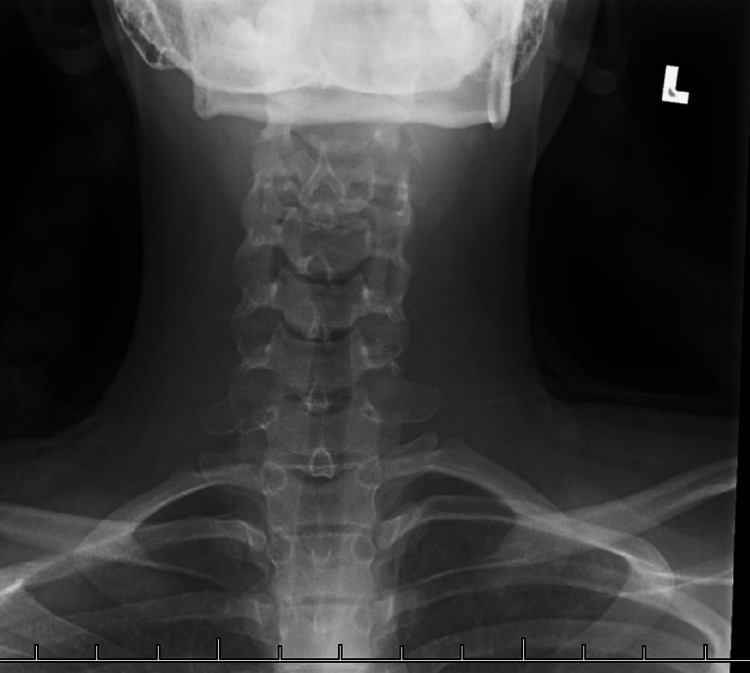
Cervical spine radiograph (AP view). AP: anteroposterior
